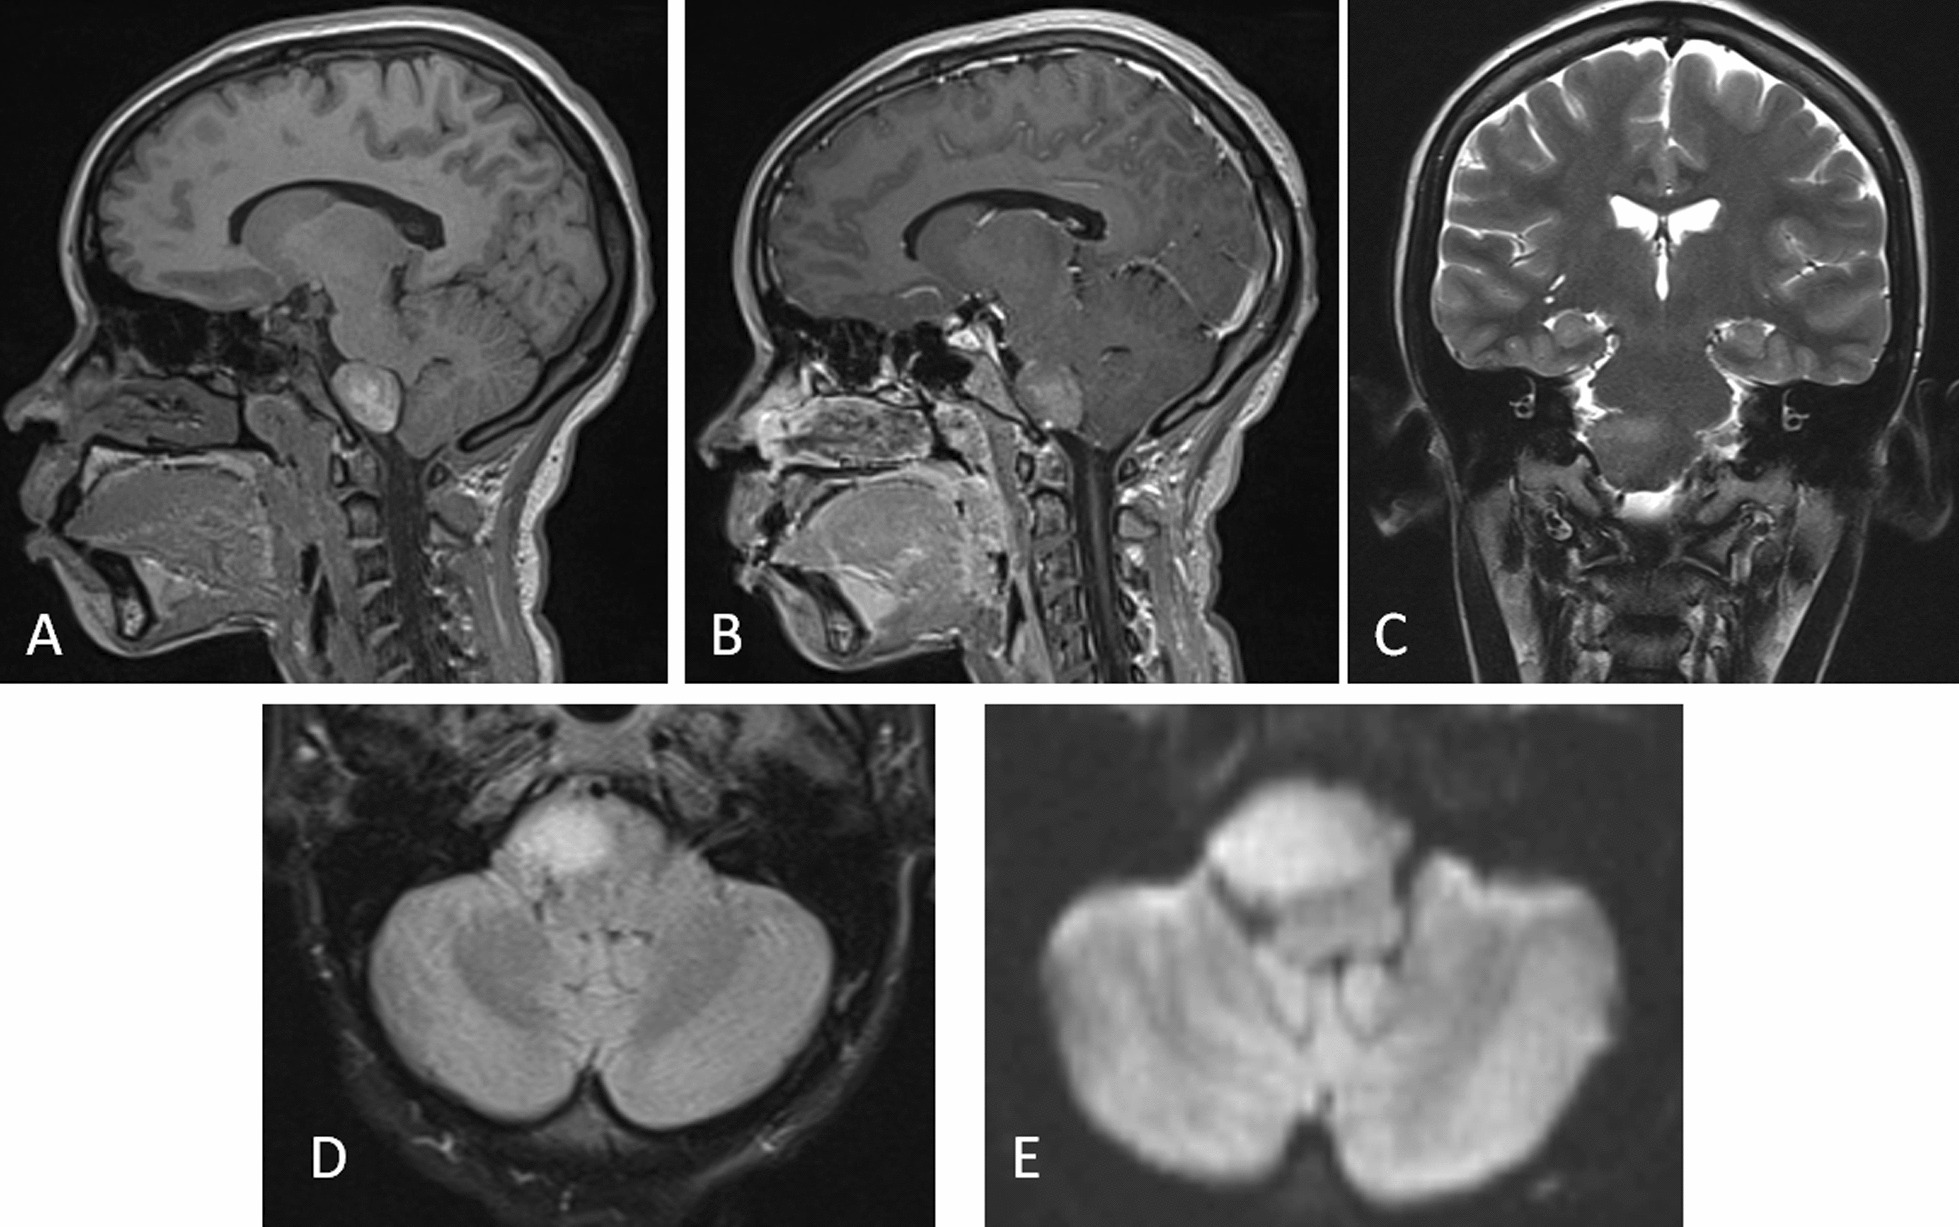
Brain MRI with contrast. A Sagittal T1 reconstruction with persistence of prepontine extraaxial mass that, when compared with previous MRI, had increased in size proportionally to the rest of the head and presented with heterogeneous signal intensity due to protein and lipid component that was not previously visualized. B Sagittal T1 contrast-enhanced sagittal reconstruction. The mass does not show enhancement after medium contrast administration. C Coronal T2 heterogeneous mass predominantly hypointense; it no longer preserves CSF-like signal intensity. D Axial FLAIR section with heterogeneity in signal intensity, although predominantly hyperintense. E Axial slice on DWI showing persistence of bright mass
